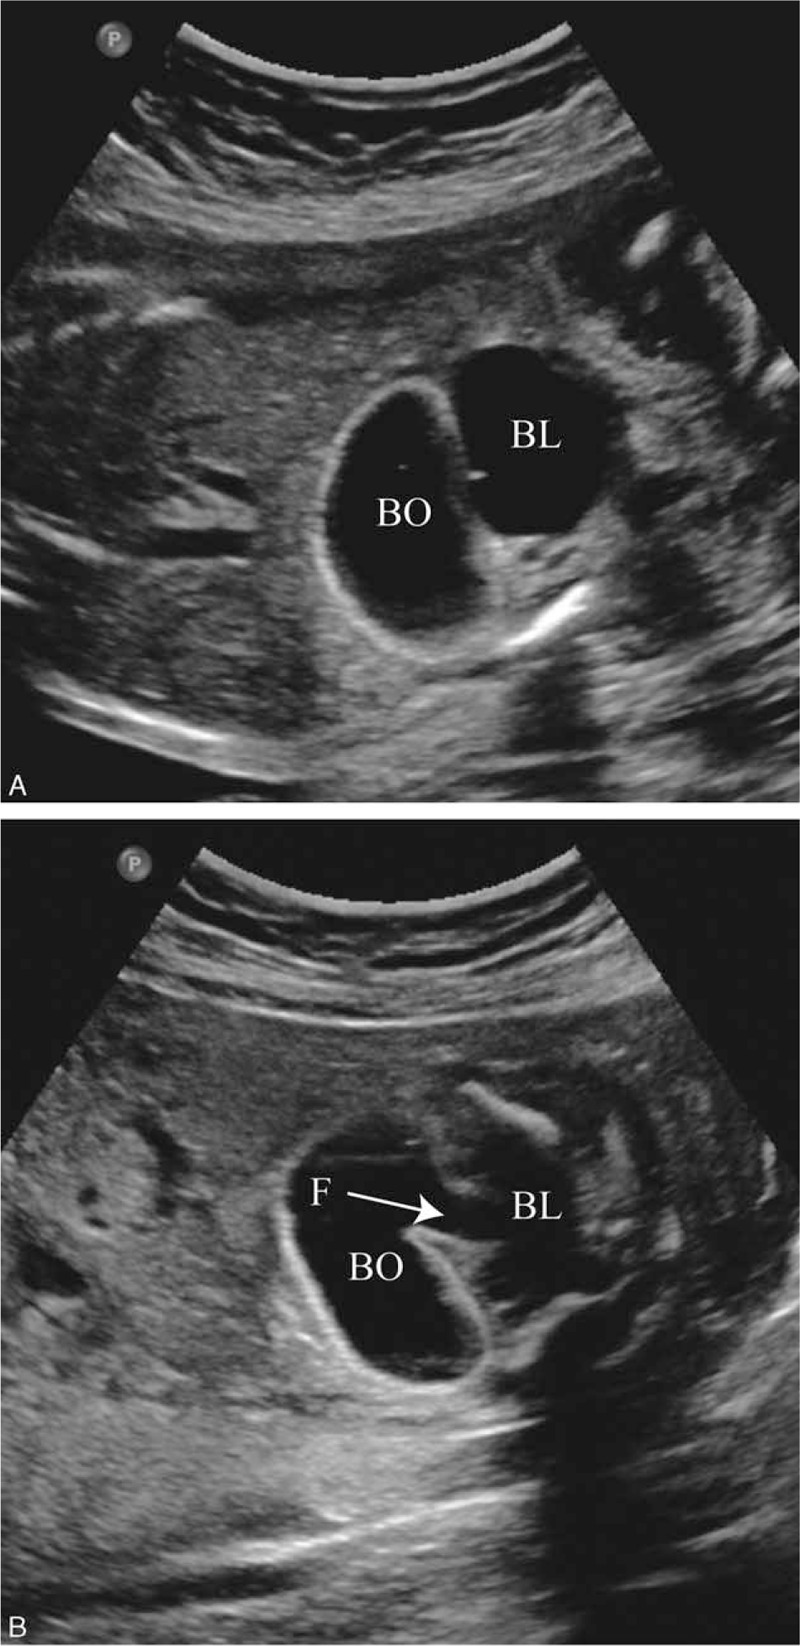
The ultrasound examination of urorectal septum malformation sequence. A, It shows distended bowel and bladder in the lower abdomen. B, The fistula is seen between bowel and bladder. BL = bladder, BO = bowel, F = fistula.
